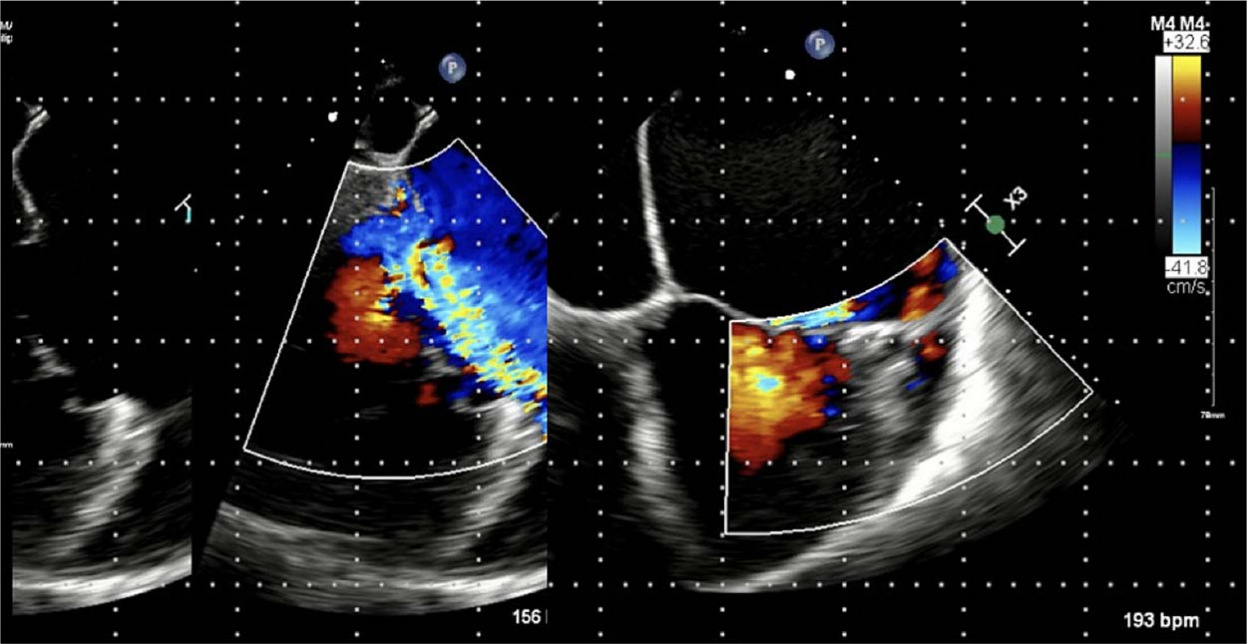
Pre-operative trans-oesophageal echocardiogram (TOE).
